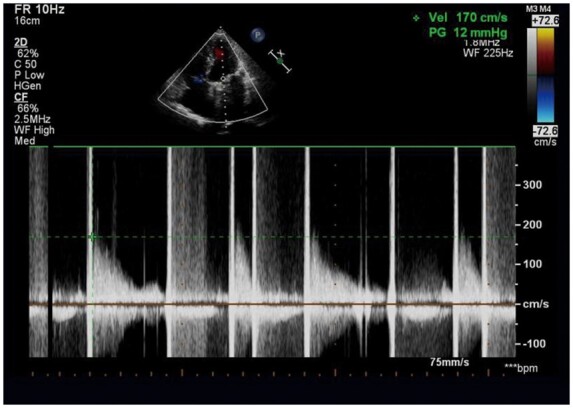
Transthoracic echocardiography showing a normal-functioning mechanical mitral prosthesis.
